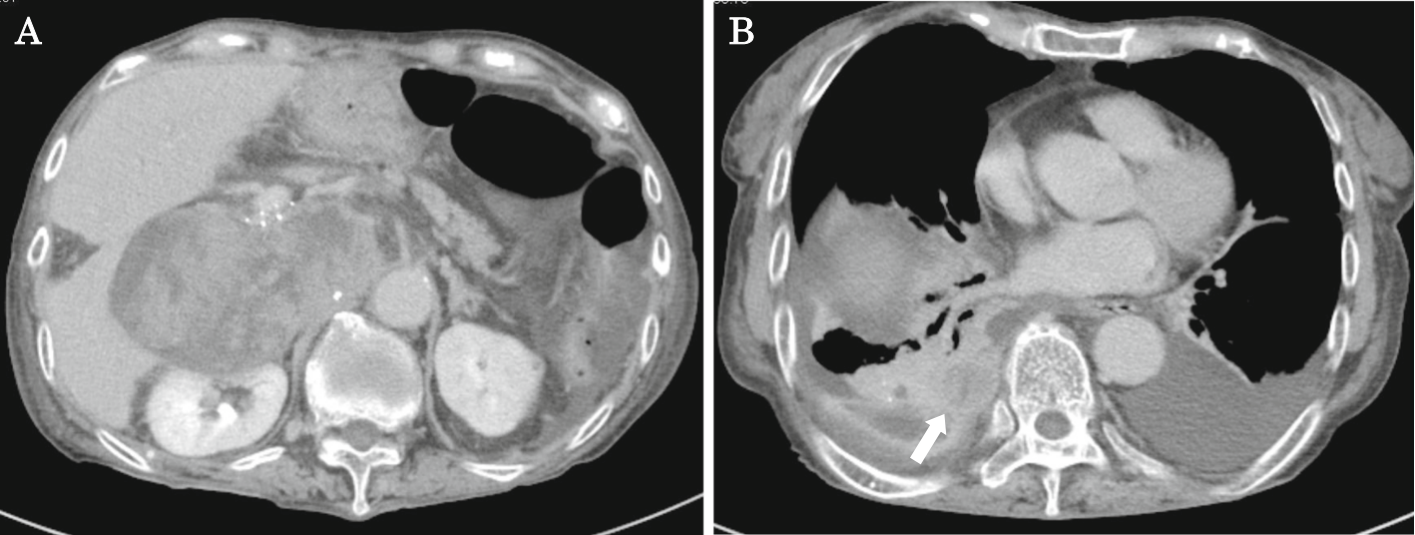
Computed tomography showed 8-cm low-density tumor compressing the inferior vena cava in the (a) liver resection area and (b) peritoneal space (arrow)
